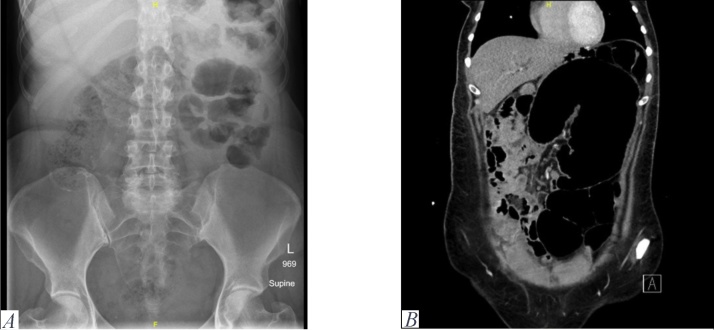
Radiological studies. A) Abdominal X-ray image showing dilated intestinal loops on the left side. B) Abdominal computed tomography image showing a 14-cm dilatation of the cecum occupying the left upper quadrant of the abdomen.
